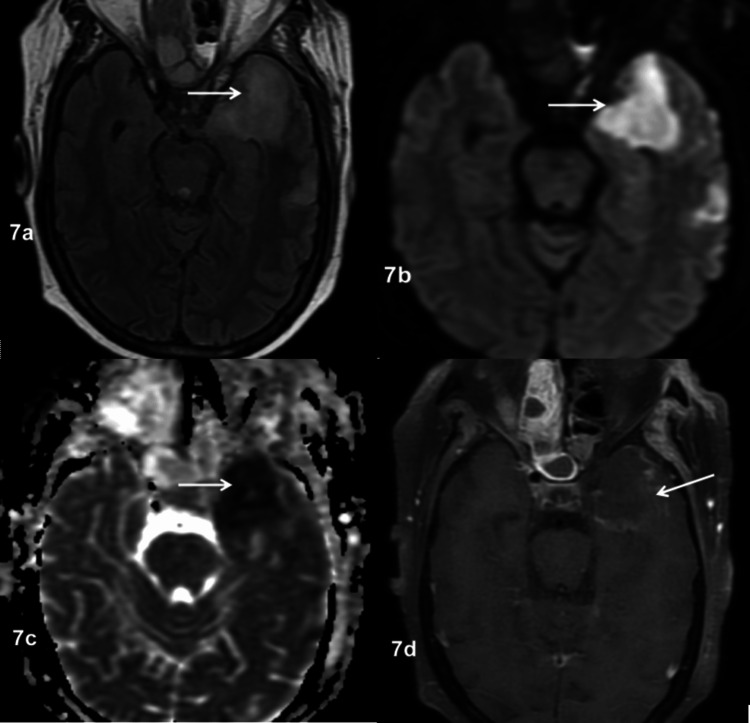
Subacute infarct in a patient with ROCM 7a: Axial FLAIR image showing hyperintensity in the left frontal lobe (arrow). 7b: Axial DWI at b-1000 showing diffusion restriction in the left frontal lobe (arrow). 7c: ADC map showing hypointensity in the corresponding region (arrow). 7d: Axial post-contrast FS T1WI showing mild peripheral enhancement, suggestive of a subacute infarct (arrow). ROCM: rhino-oculo-cerebral mucormycosis, FLAIR: fluid-attenuated inversion recovery, DWI: diffusion-weighted imaging, ADC: apparent diffusion coefficient, FS T1WI: fat-suppressed T1-weighted image
